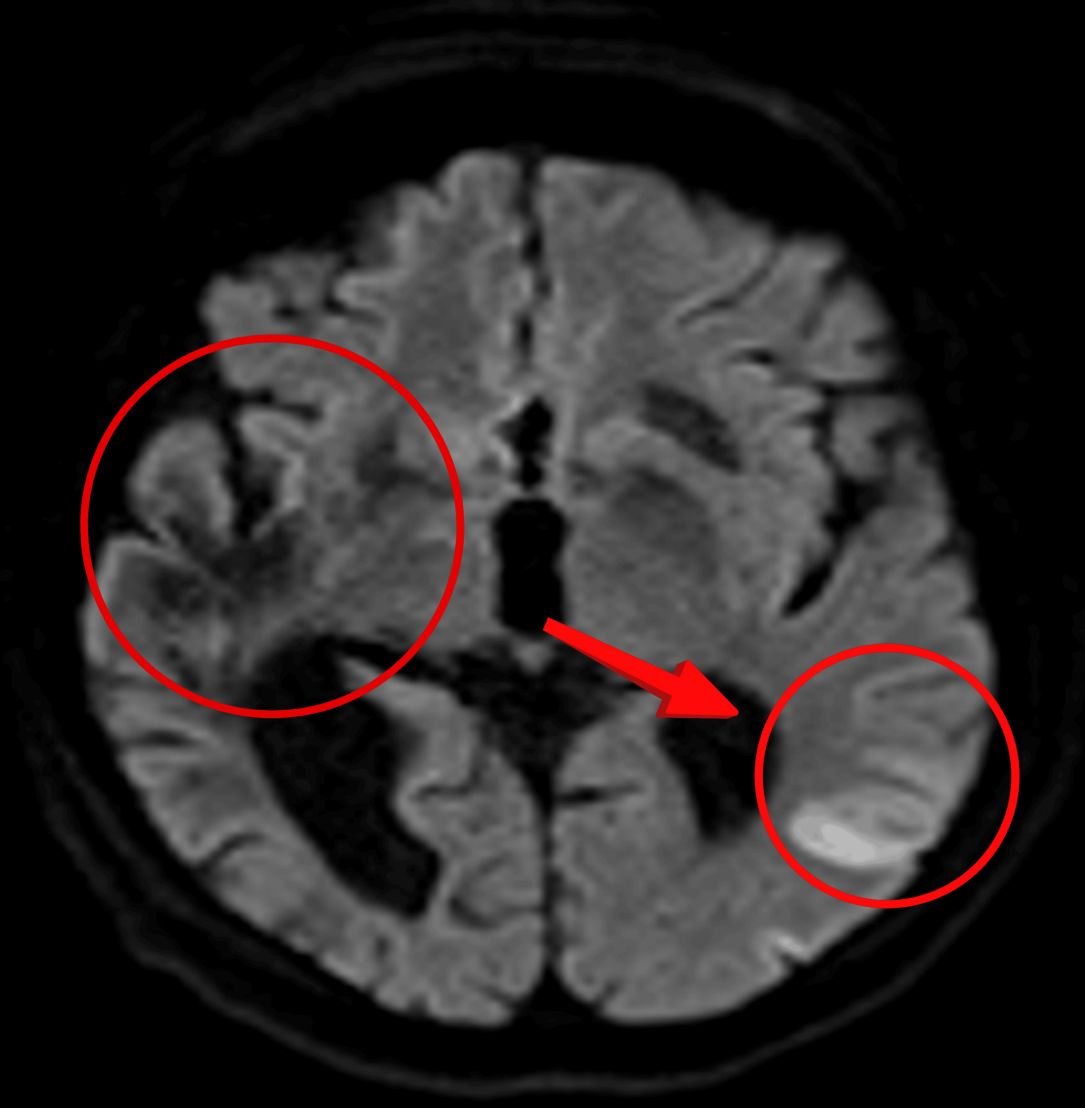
MRI head showing acute infarct in the left MCA territory and an old infarct in the right MCA territory MRI: magnetic resonance imaging; MCA: middle cerebral artery
